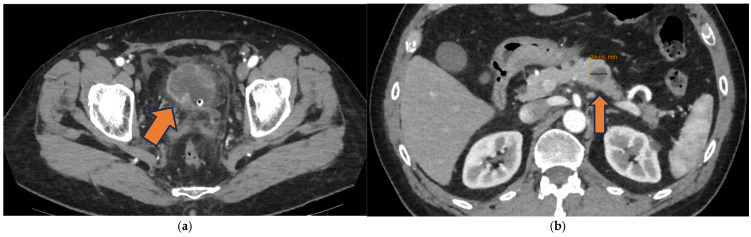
Radiologic findings upon contrast-enhanced CT scan of October 2025: (a) On the right bladder wall, a pathological, cauliflower-like thickening is noted, with rim enhancement and an apparently predominantly extravisceral growth pattern; (b) The 1.5 cm hypodense nodule in the pancreatic body, with irregular margins and minimal contrast enhancement.
